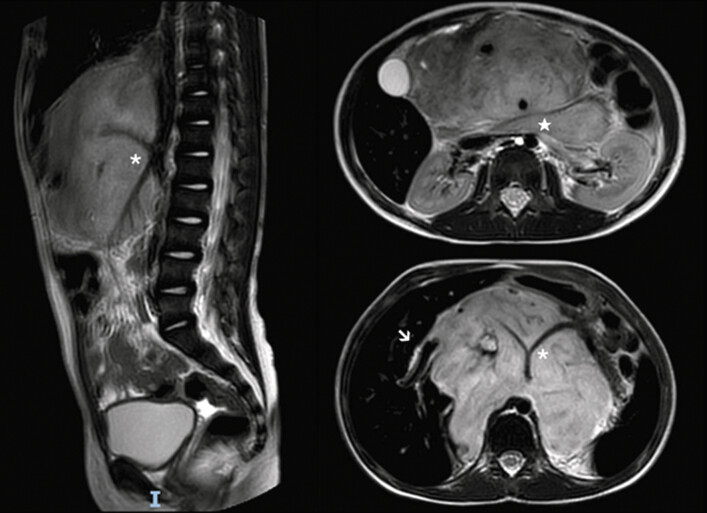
Preoperative Magnetic Resonance Imaging showing tumor infiltration of the porta hepatis (arrow), tumor encasement of the origins of the celiac axis and the superior mesenteric artery (asterisk), and tumor infiltration of the duodenopancreatic region (star).
